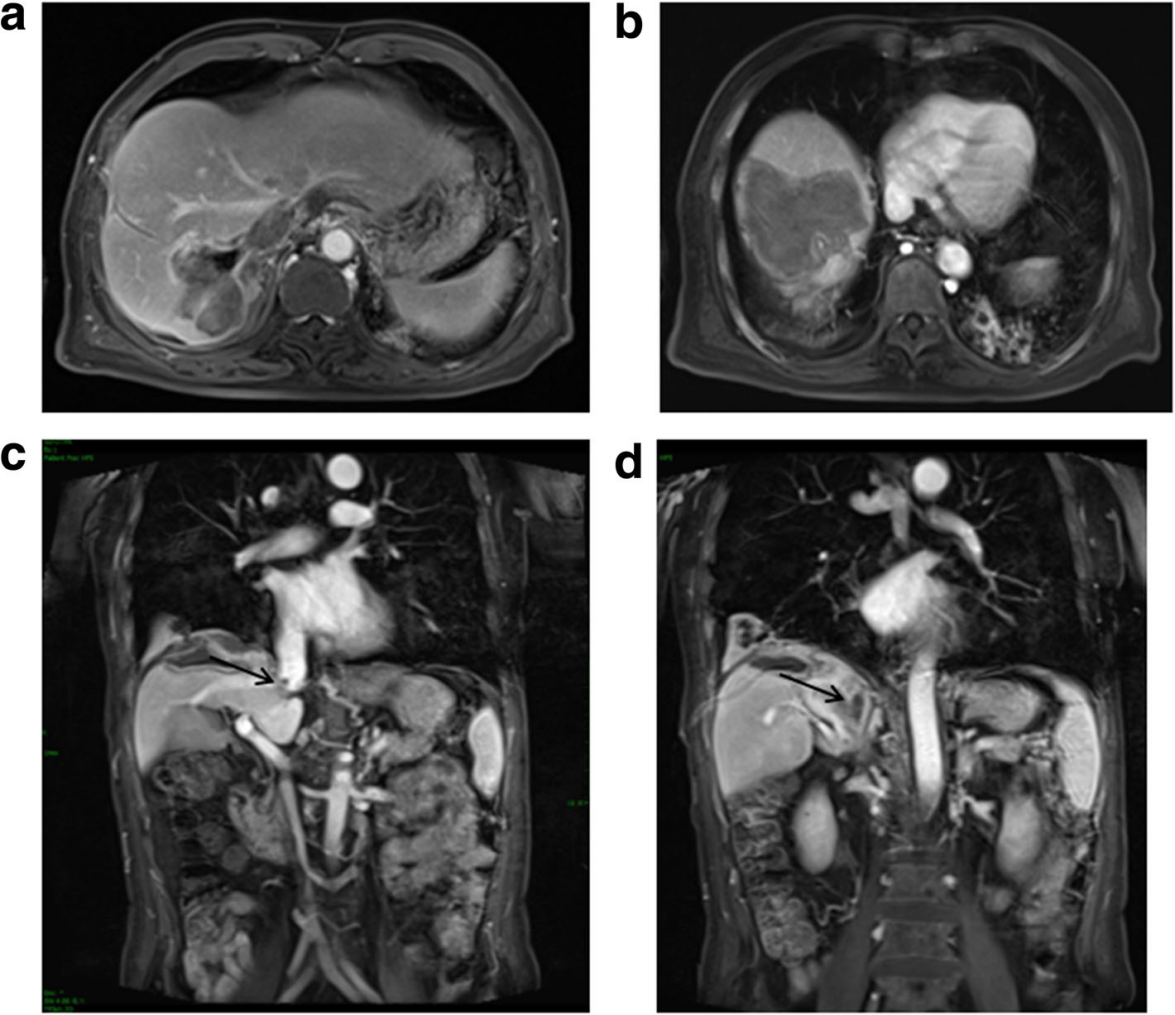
MRI at 5 month post-operatively. a, b Enhanced MRI of the abdominal region showed further shrunk ablated tumor area at segment VII and VIII. c, d Coronal sequence of enhanced MRI of the abdominal region showed the further shrinkage of the tumor thrombus (*black arrow*). MRI: magnetic resonance imaging, RA: right atrium, IVC: inferior vena cava
